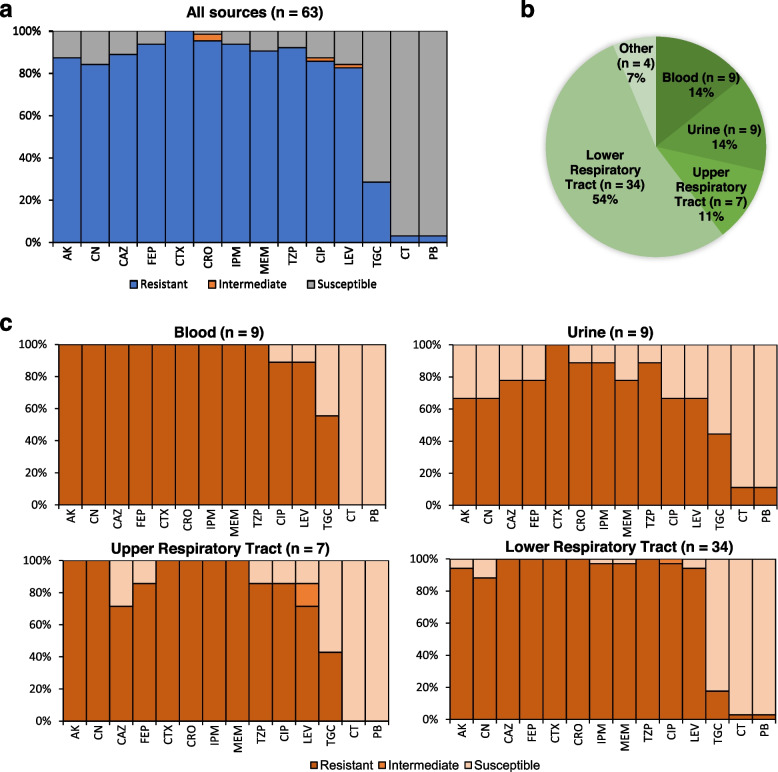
a. Antimicrobial susceptibility profile of 63 ACB complex strains against 14 antibiotics. b. Source distribution of 63 ACB complex strains c. Source-wise antimicrobial susceptibility profiles of the 63 ACB complex strains. AK = Amikacin; CN = Gentamicin; CAZ = Ceftazidime; FEP = Cefepime; CTX = Cefotaxime; CRO = Ceftriaxone; IPM = Imipenem; MEM = Meropenem; TZP = Tazobactam/Piperacillin; CIP = Ciprofloxacin; LEV = Levofloxacin; TGC = Tigecycline; CT = Colistin, PB = Polymyxin B
